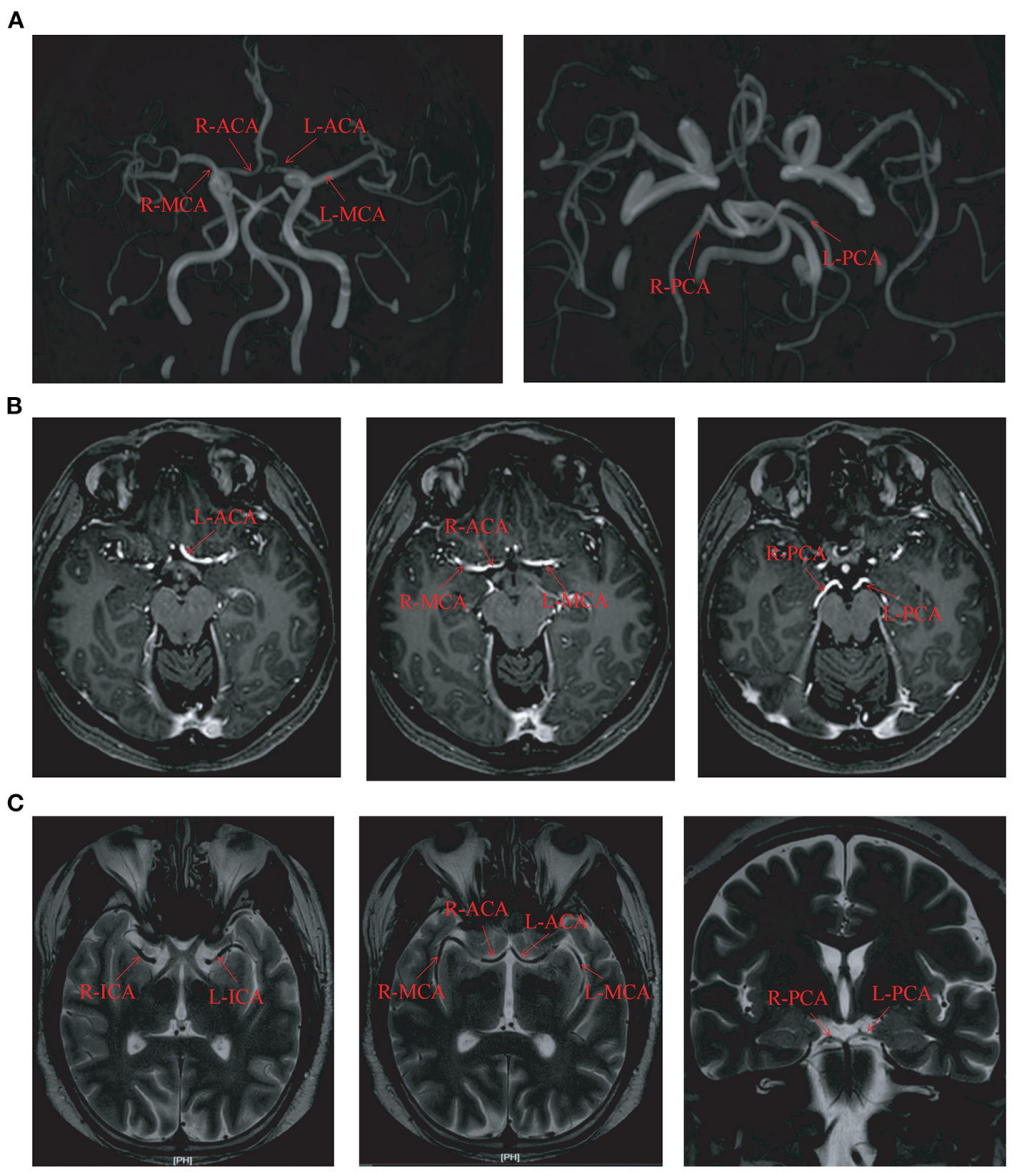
The circle of Willis imaging in some cases. (A) The magnetic resonance angiography imaging in case number 3. (B) The hippocampal magnetic resonance imaging in case number 2. (C) The thin-layer enhanced MRI imaging in case number 15. L, left; R, right; ACA, anterior cerebral artery; MCA, middle cerebral artery; PCA, posterior cerebral artery; ICA, Internal carotid artery.
